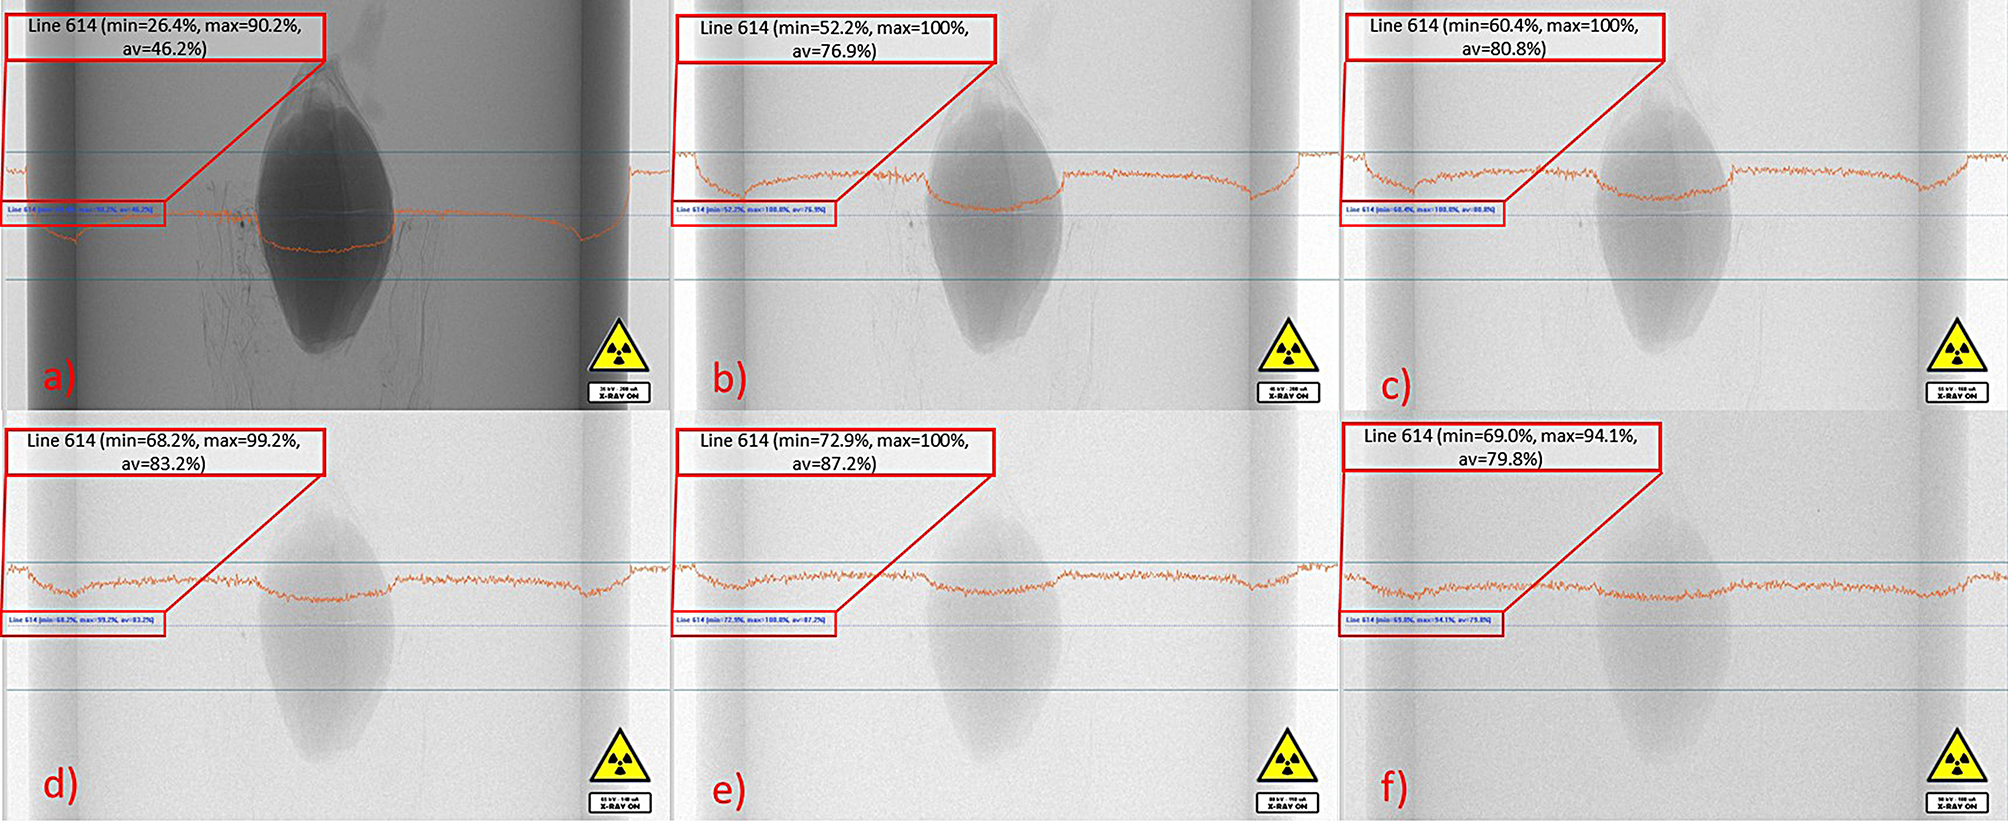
A comparison of possible filters available on the SkyScan 1272, each image shows the flat field, highlighting the maximum, minimum and average attenuation of the x-ray as it passes through the widest part of the sample. (a) no filter, (b) 0.25 mm aluminum, (c) 0.5 mm aluminum, (d) 1 mm aluminum, (e) 0.035 mm copper and 1 mm aluminum, (f) 0.11 mm copper
